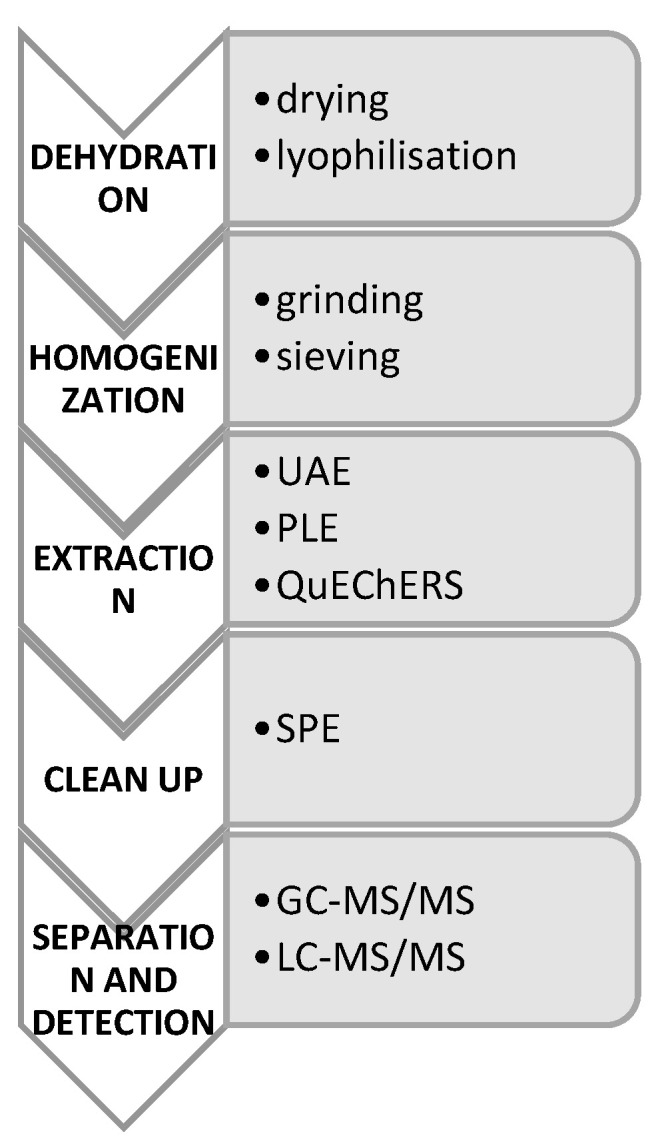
Stages of the analysis of the content of micropollutants in sewage sludge with examples of methods [11,19,20,22]. Abbreviations: UAE—ultrasound-assisted extraction; PLE—pressurised liquid extraction; QuEChERS—quick, easy, cheap, effective, robust, and safe; SPE—solid-phase extraction; GC—gas chromatography; LC—liquid chromatography; MS/MS—tandem mass spectrometry.
